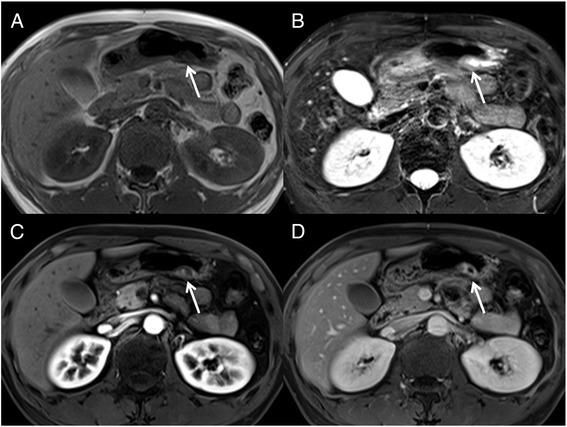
Magnetic resonance imaging (MRI) (a, T1-weighted image; b, fat suppressed T2-weighted image; c, arterial phase; d, delayed phase) of the tumor showed cysts with a thin wall. The wall exhibited hyperintensity on T2-weighted images and a gradual enhancement pattern, which reflects the myxoid nature of the tumor
